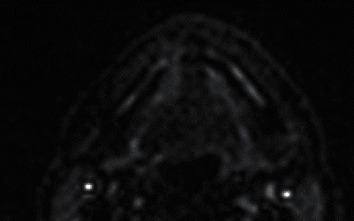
Magnetic resonance imaging, horizontal cross section, short T1 inversion recovery. There are no signs of tumors or inflammation.
